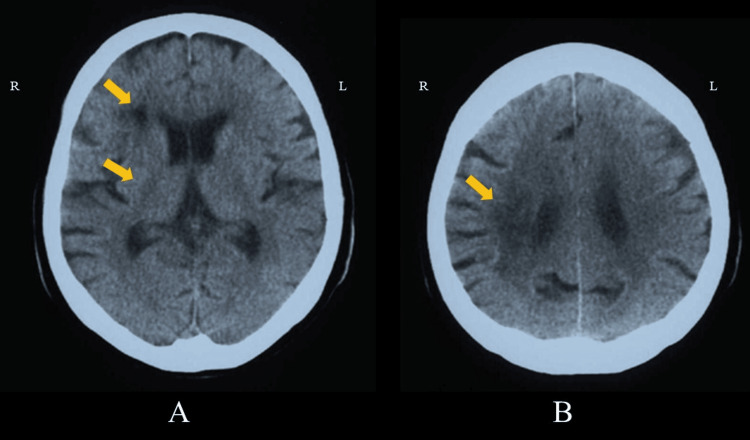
Brain CT (Case 2). Brain CT revealing a low-density area in the right middle cerebral artery territory, including putamen, corona radiata, and centrum semiovale (arrow): (A) basal ganglia level slice view and (B) semioval center level slice view. R: right side, L: left side, CT: computed tomography.
